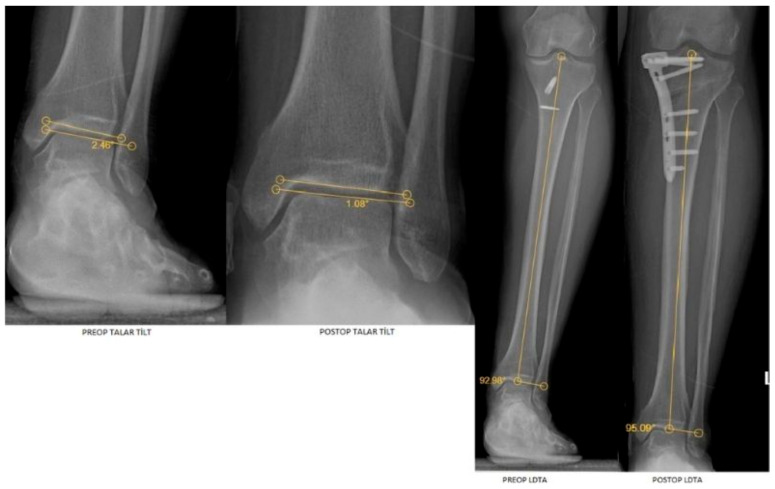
Preoperative and postoperative radiographic images demonstrating ankle joint measurements, including talar tilt and lateral distal tibial angle (LDTA).
